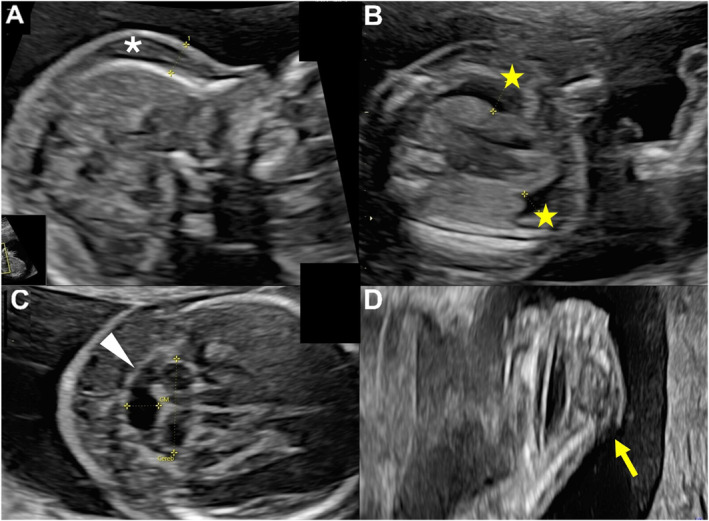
Second‐trimester ultrasound imaging. (A) Prefrontal edema measuring 6.4 mm (white asterisk). (B) Bilateral pleural effusion (yellow stars) measuring 6.3 and 4.56 mm respectively. (C) Transcerebellar diameter below 1% for gestational age (white arrowhead). (D) Congenital vertical talus (yellow arrow).
